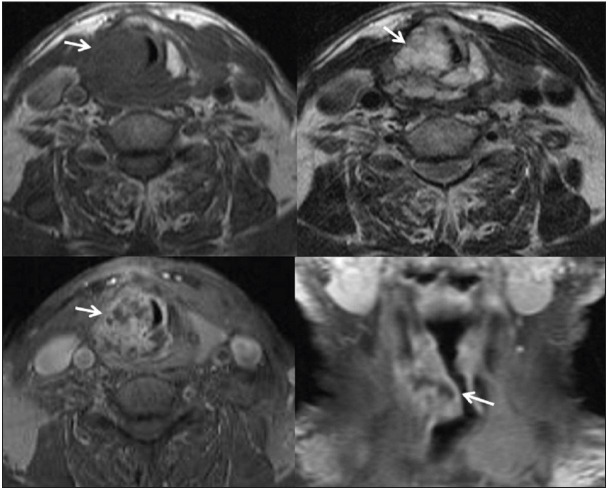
In axial and coronal MRI images, a malignant mass invading thyroid, arytenoid and cricoid cartilages (arrows).
